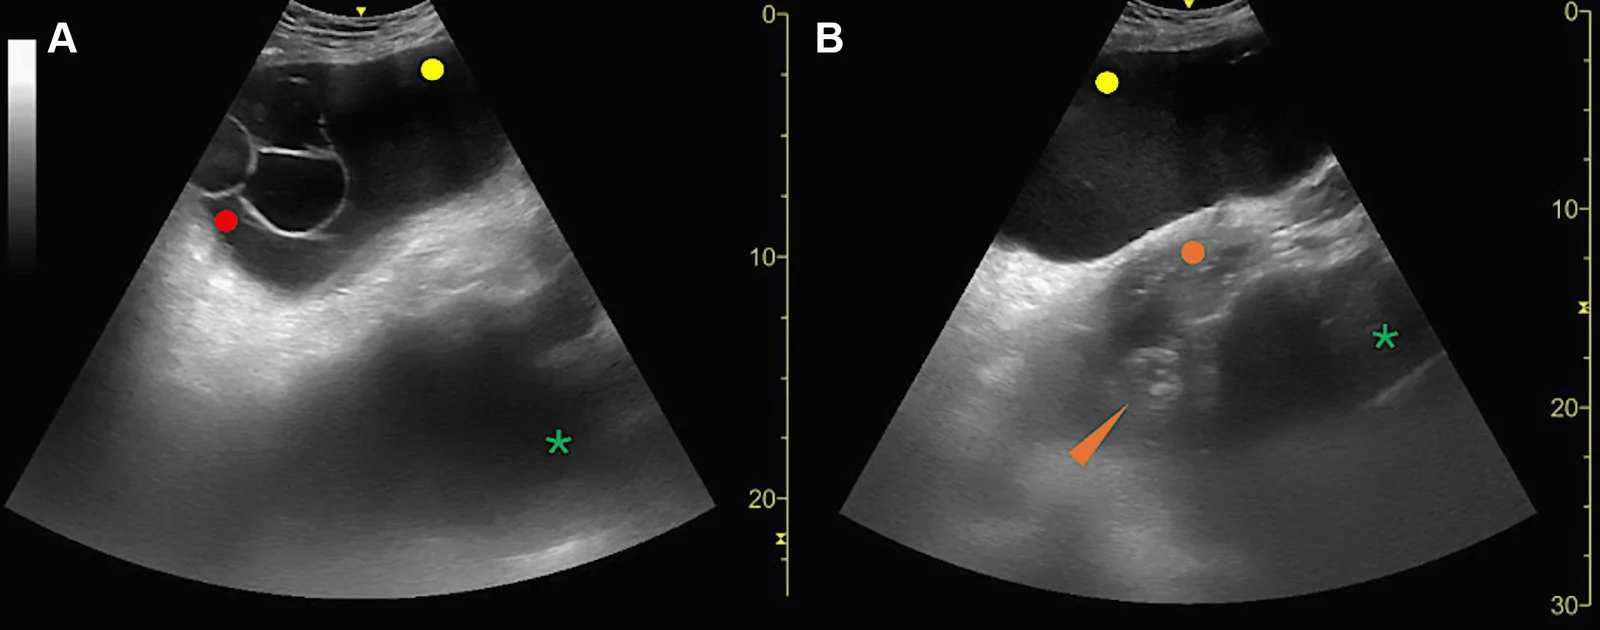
Bedside point-of-care ultrasound image with a low-frequency curvilinear probe in sagittal orientation, visualizing (A) anechoic, fluid-filled structure (yellow circles) with an internal cystic mass with septations (red circle) and (B) a Foley catheter (orange arrowhead) in a collapsed bladder (orange circle). Bowel is visualized deep to the fluid-filled structure and bladder (green asterisks).
